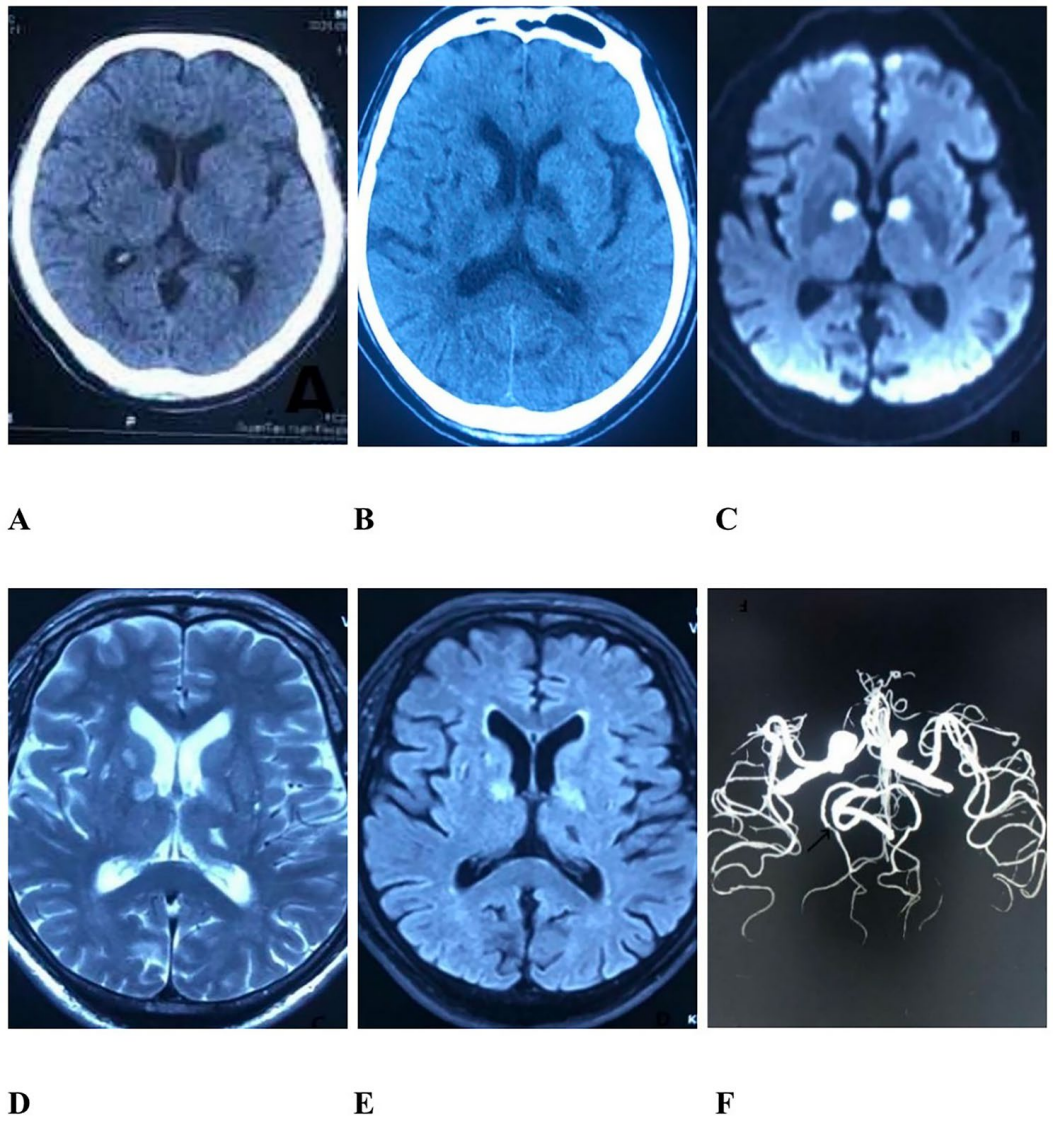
The patient’s imaging results (A) Head CT showed no obvious abnormalities (September 11, 2020). (B) Head CT showed bilateral low-density lesions in the anterior thalamus (September 12, 2020). (C-E) DWI, T2 weighted imaging, and FLAIR of the head showed symmetrical bilateral high signal lesions in the anterior part of the thalamus (September 12, 2020). (F) CTA of the head showed moderate to severe local stenosis of the P3 segment of the left posterior cerebral artery (the black arrow)
